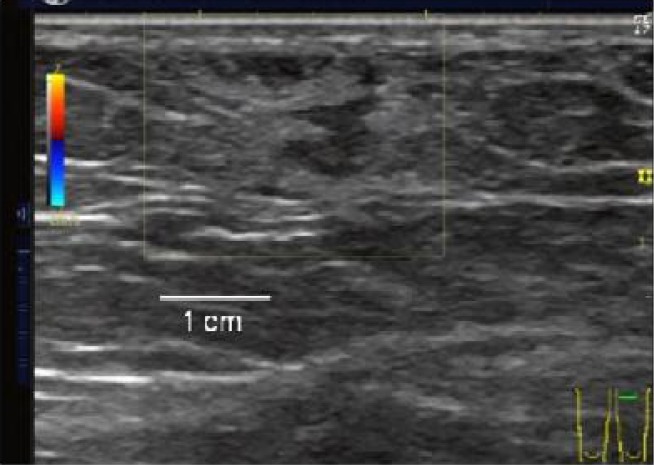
Sparganosis. Ultrasonography shows a serpiginous hypoechoic structure and this compartment is surrounded by a hyperechoic area
